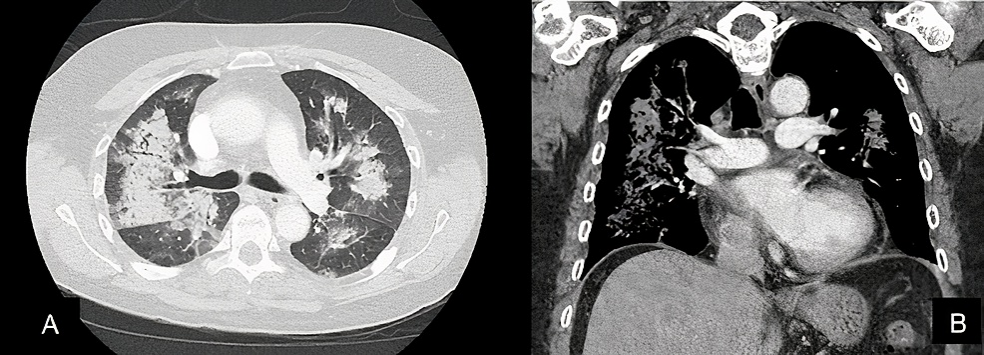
A-B. CT chest displaying diffuse consolidation throughout lung fields.
